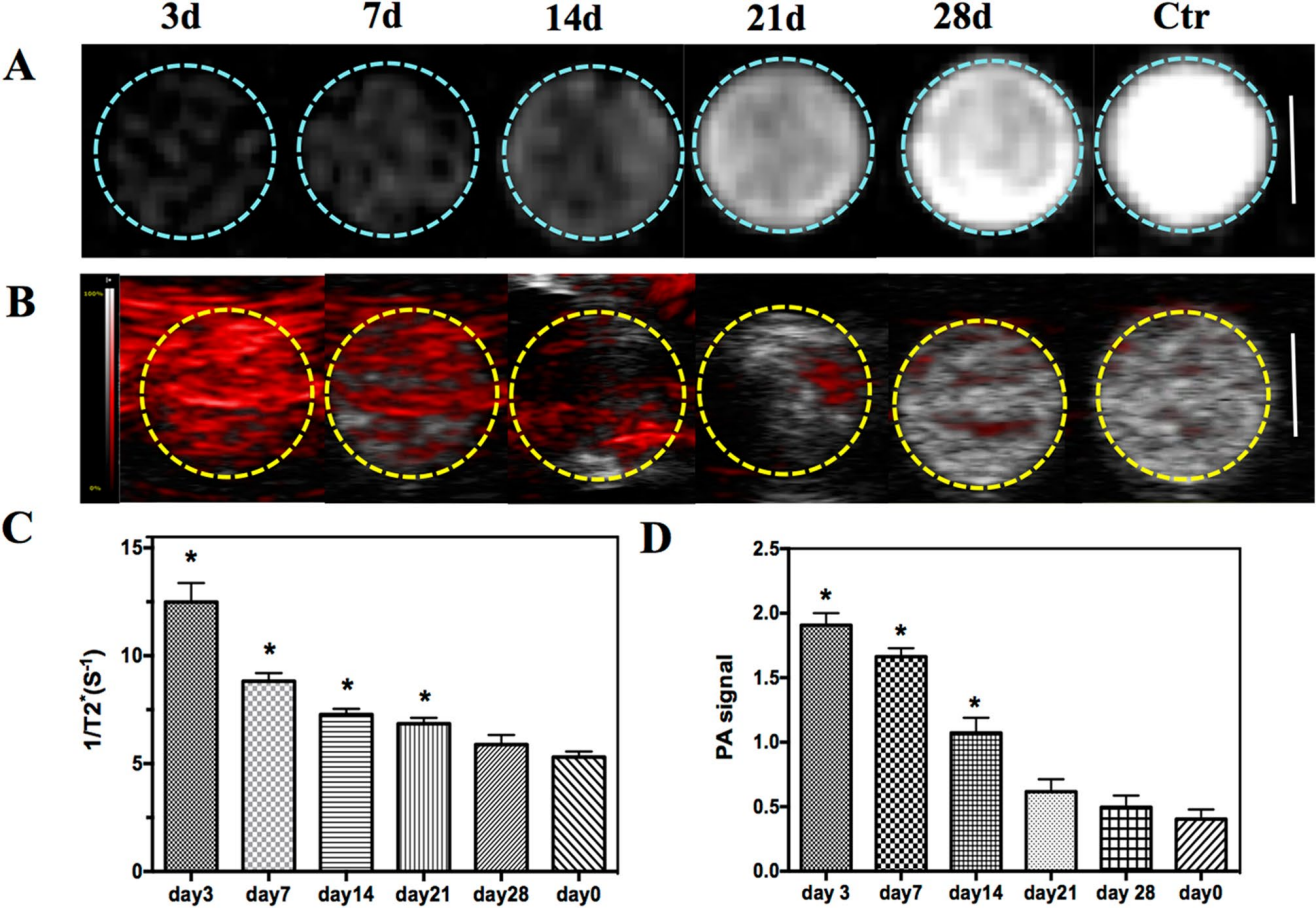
Efficiency of PLGA/IO MPs for long-term tracking of TSCs in vitro. A T2^*^-weighted MR images of labeled TSCs at different time point and unlabeled TSCs (Ctr) in agarose gel. Scale bar = 5 mm. B PA images of labeled TSCs at different time point and unlabeled TSCs (Ctr) in agarose gel. Scale bar = 2 mm. C The intensity of PA signal value of labeled TSCs at different iron concentrations by comparison with unlabeled TSCs (Day 0). D The intensity of MR signal value of labeled TSCs at different iron concentrations by comparison with unlabeled TSCs (Day 0). ^*^, *P* < 0.05.
